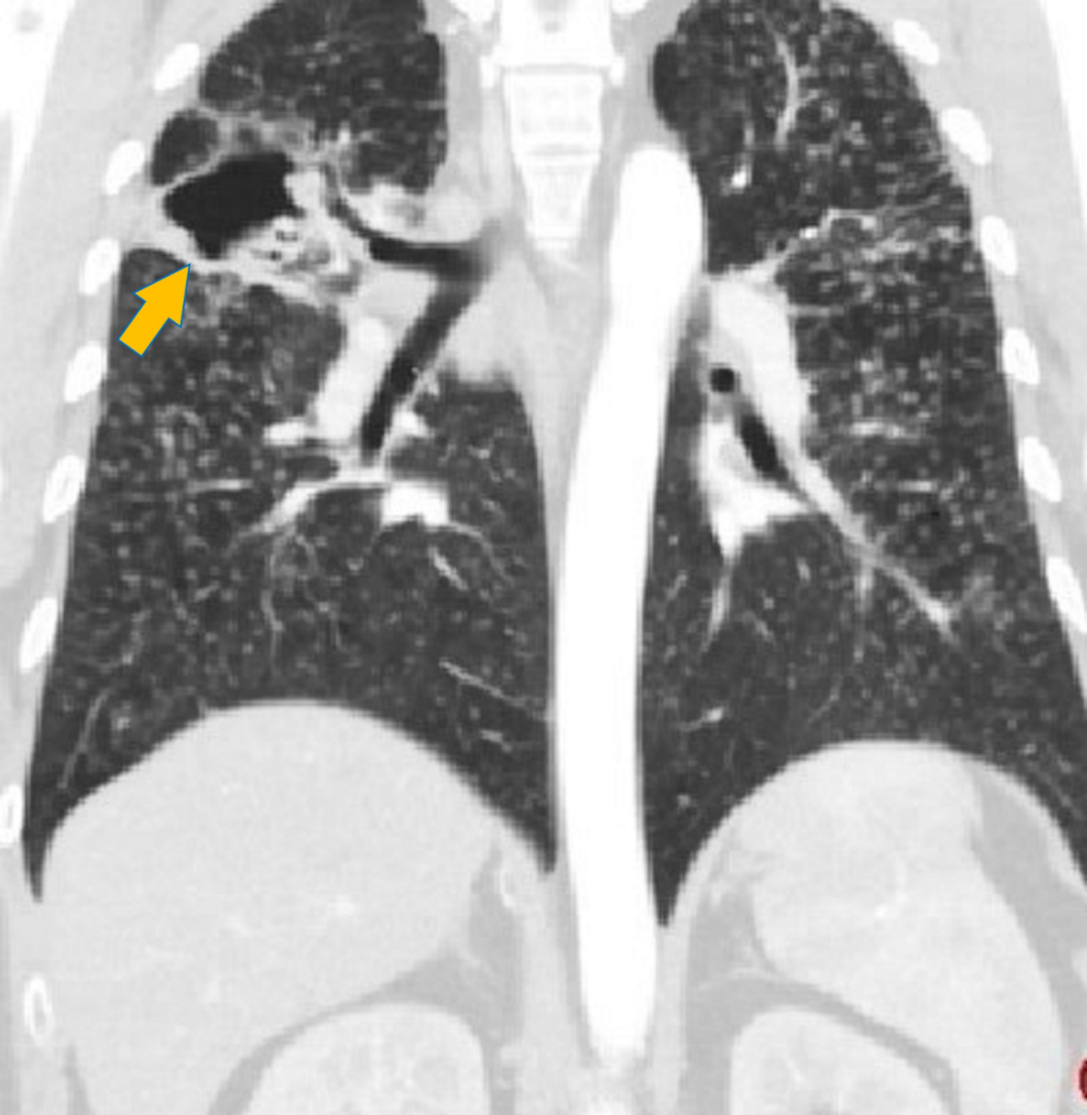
Computed tomography scan of the chest revealing a 4.7-cm right upper lobe cavitary lesion and multiple bilateral nodules in a miliary pattern throughout the lungs with mediastinal lymphadenopathy.
